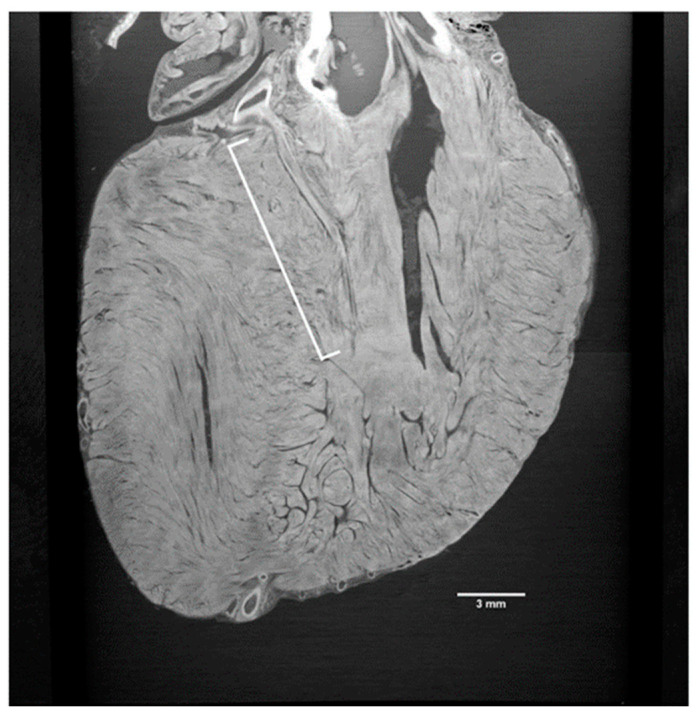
X-ray phase-contrast tomography image displaying the initial septal branch of the left anterior descending artery (LAD); here, a two-dimensional depiction of the heart is captured from a distinct angle. The image distinctly showcases the section of the first septal branch (highlighted by the white bracket) stemming from the LAD (CC-BY 4.0) [26].
